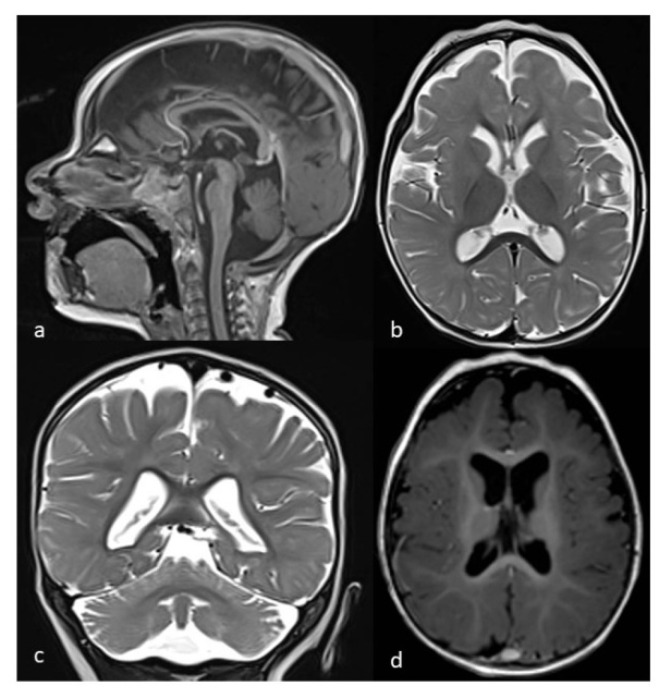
Brain MRI (T1-weighted in (a,d); T2-weighted in (b,c)) of the patient performed at age 14 months showing thin corpus callosum and hypoplasia of pons (a) and cerebellar vermis (a,c), simplified gyral pattern with reduction in frontal gyri and enlargement of sub-arachnoids spaces (b,d), mild reduction in the total amount of white matter (b,d) and enlargement of cerebellar interfolial spaces (c).
